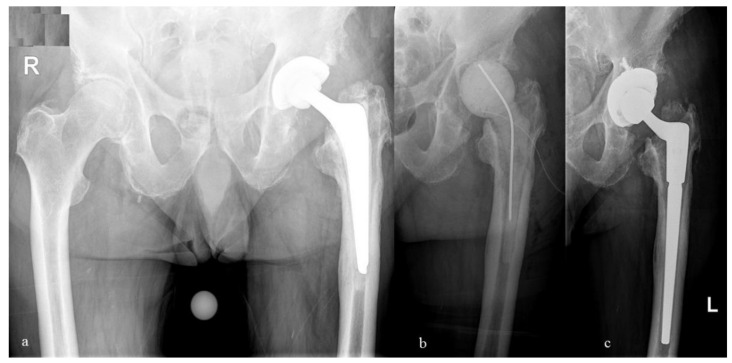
(a) Anterio-posterior radiographs of the pelvis of an 86-year-old man with a septic loosening of the femoral stem; (b) Following prosthesis explantation, an articulating spacer was inserted into the femur; (c) After an interim period of 69 days, the prosthesis reimplantation was performed with cementless implants (Restoration Acetabular Shell^®^, Restoration Stem^®^, Fa. Stryker, Duisburg, Germany).
